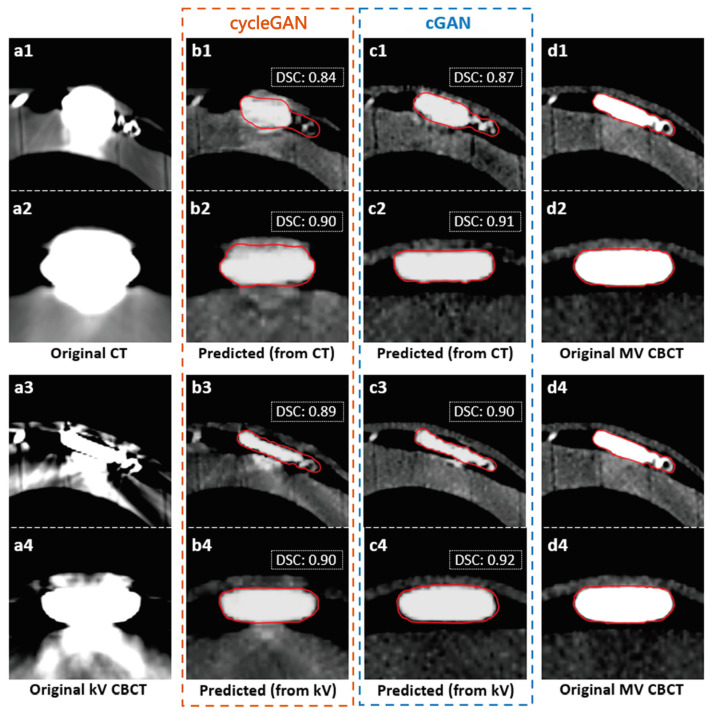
Comparison between the contours on synthetic and real MV CBCT imagesRed lines indicate the pacemaker contours delineated and reviewed by three users. The Dice similarity coefficient (DSC) results comparing the contours between the original and synthetic images are included for reference.
